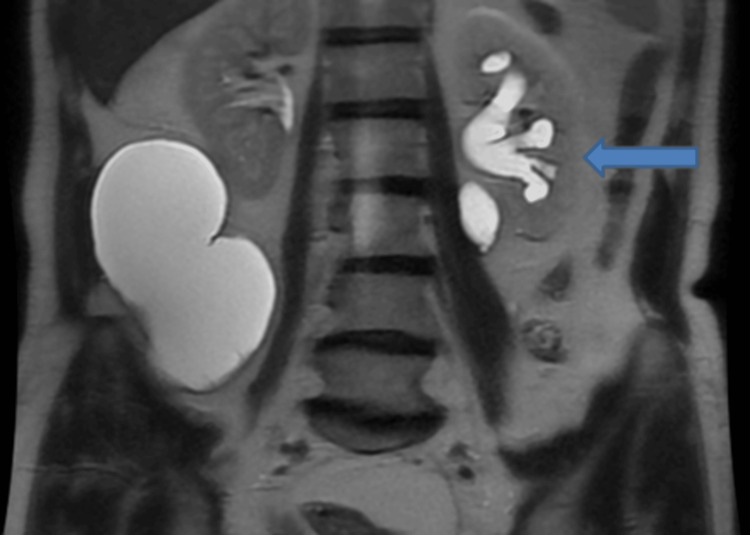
Non-contrast T2-weighted MRI of the abdomen (coronal view) showing dilatation of the left renal collecting system (blue arrow).
